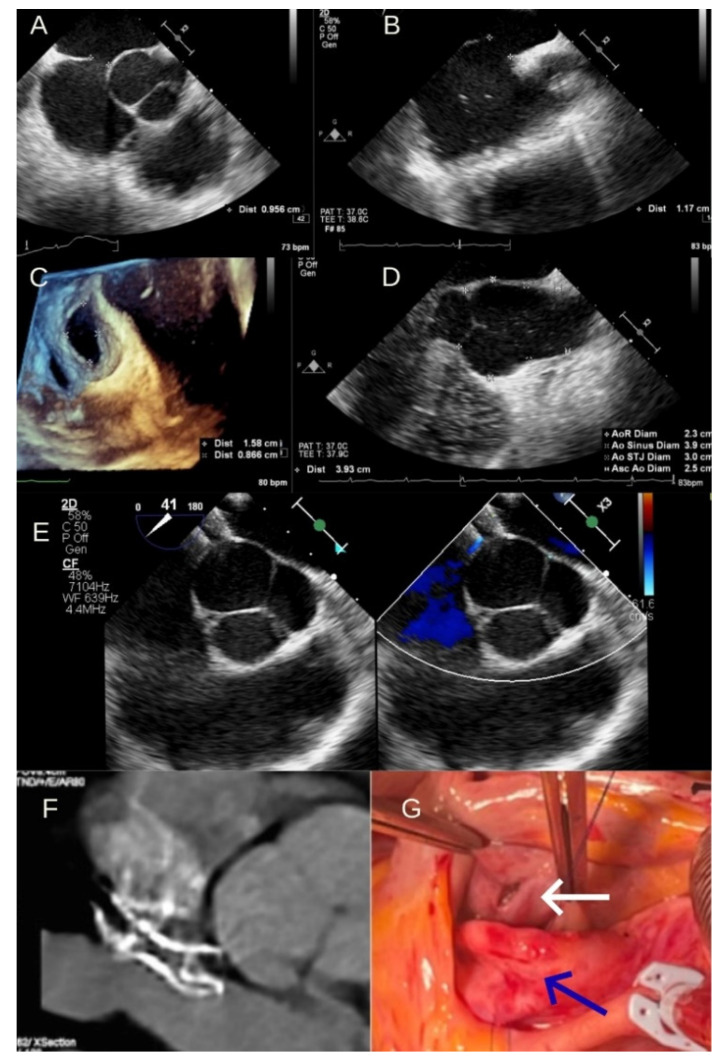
(A). 2D TEE evaluation short axis view showing 9.5 mm OS ASD, deficient aortic rim; (B). TEE evaluation bicaval view showing an 11.7 mm OS ASD, good inferior and superior rim; (C). 3D zoom TEE evaluation showing a 15/8.6 mm oval ASD, deficient aortic rim; (D). 2D TEE evaluation long axis view showing mild dilation of the aorta at Valsalva sinus (39 mm); (E). Intraprocedural 2D TEE evaluation showing ASO device oriented towards the aorta, with a configuration suggestive of potential intermittent contact between the device disk and the aortic wall; (F). Cardiac CT showing ASO device protruding through the pericardial space into the aortic root: aorto-atrial erosion; (G). Picture taken during surgery revealing complete erosion of atrial wall (ASO device visible through the erosion)—white arrow; Superficial Aortic wall erosions are also visible with only intima left intact—blue arrow.
